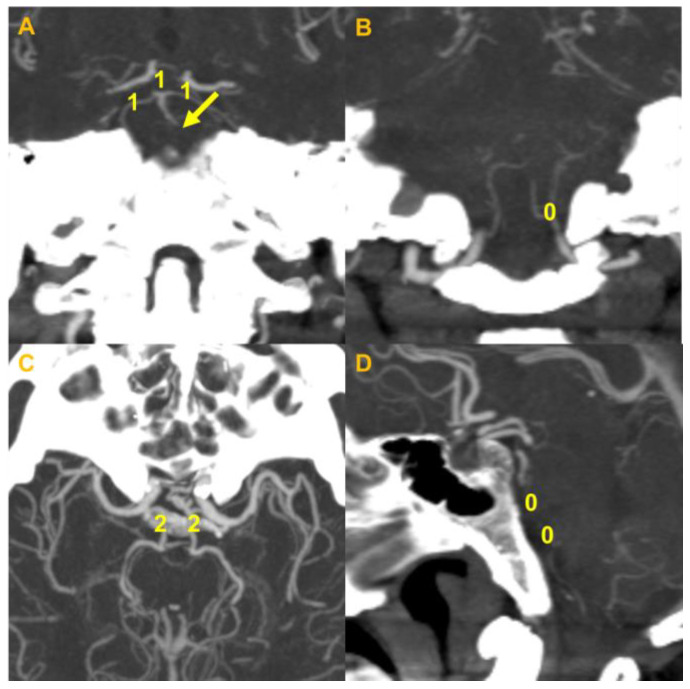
An example of a BATMAN score from a truncal-type occlusion from the cohort. (A) Coronal reconstruction of the MIP image of the basilar artery. Occlusion at the vertebrobasilar artery with visualization of the distal part and the basilar top is seen. Both PCAs are visualized. For the BATMAN score, each 1 point is given for the distal BA, and both PCAs. (B) Coronal MIP image of bilateral VAs. Note that both VAs are occluded. Zero point was given. (C) Axial MIP image showing both posterior communicating arteries. Two points for each communicating artery was given. (D) Sagittal MIP image showing occluded proximal and mid-BA. Zero points were given for each part of the BA, constituting a total BATMAN score of 7. BATMAN, Basilar Artery on Computed Tomography Angiography; BA, basilar artery; MIP, maximal intensity projection; PCA, posterior cerebral artery; VA, vertebral artery.
